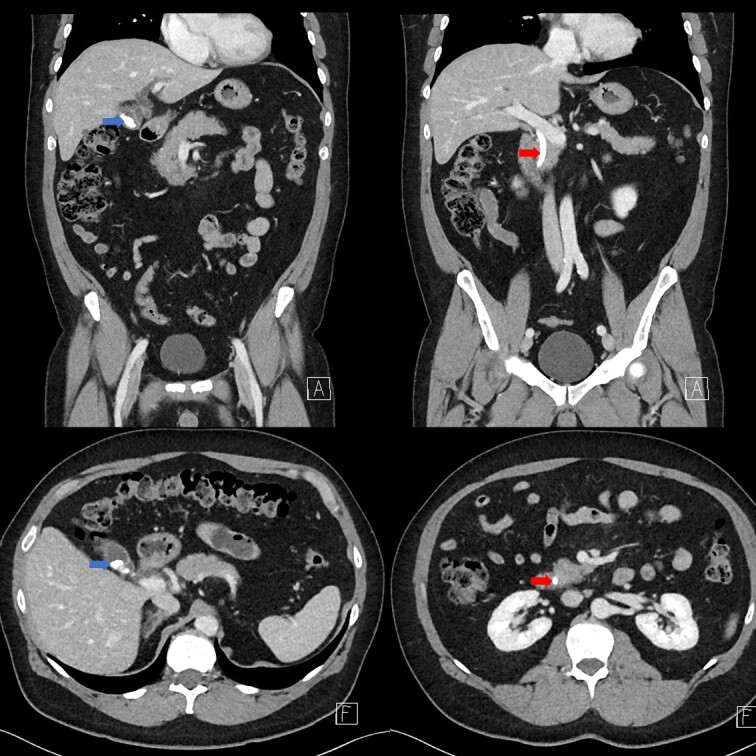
Contrast-enhanced CT demonstrating limy bile within the gallbladder neck (left image) and CBD (right image).
